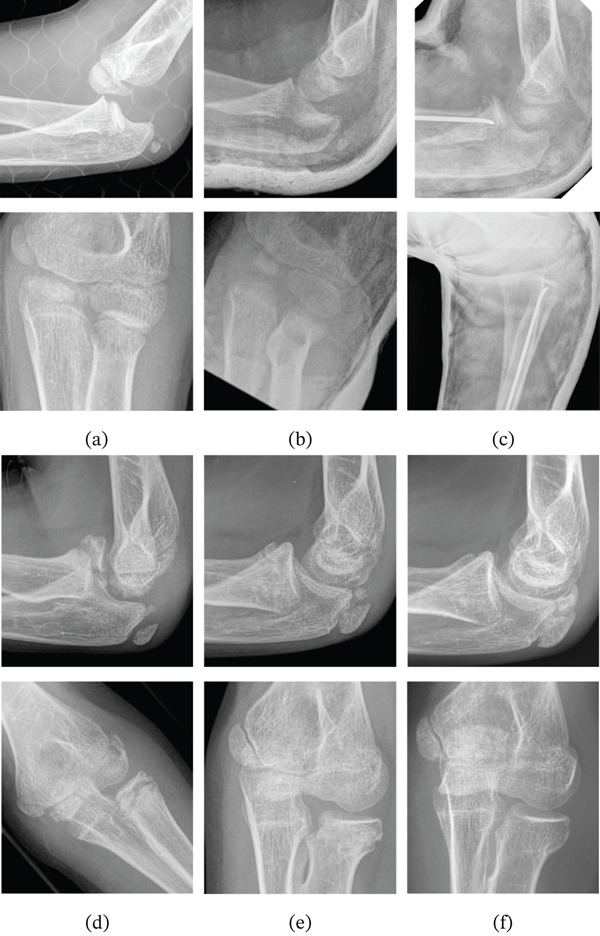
Radiographic evolution of Case 1 (lateral views in the upper row and anteroposterior views in the lower row): (a) dislocation of the left elbow, (b) Jeffery lesion after reduction, (c) open reduction and osteosynthesis with elastic nail, (d) removal of osteosynthesis material at 13 months, (e) follow‐up at 2 years and (f) follow‐up at 4 years.
